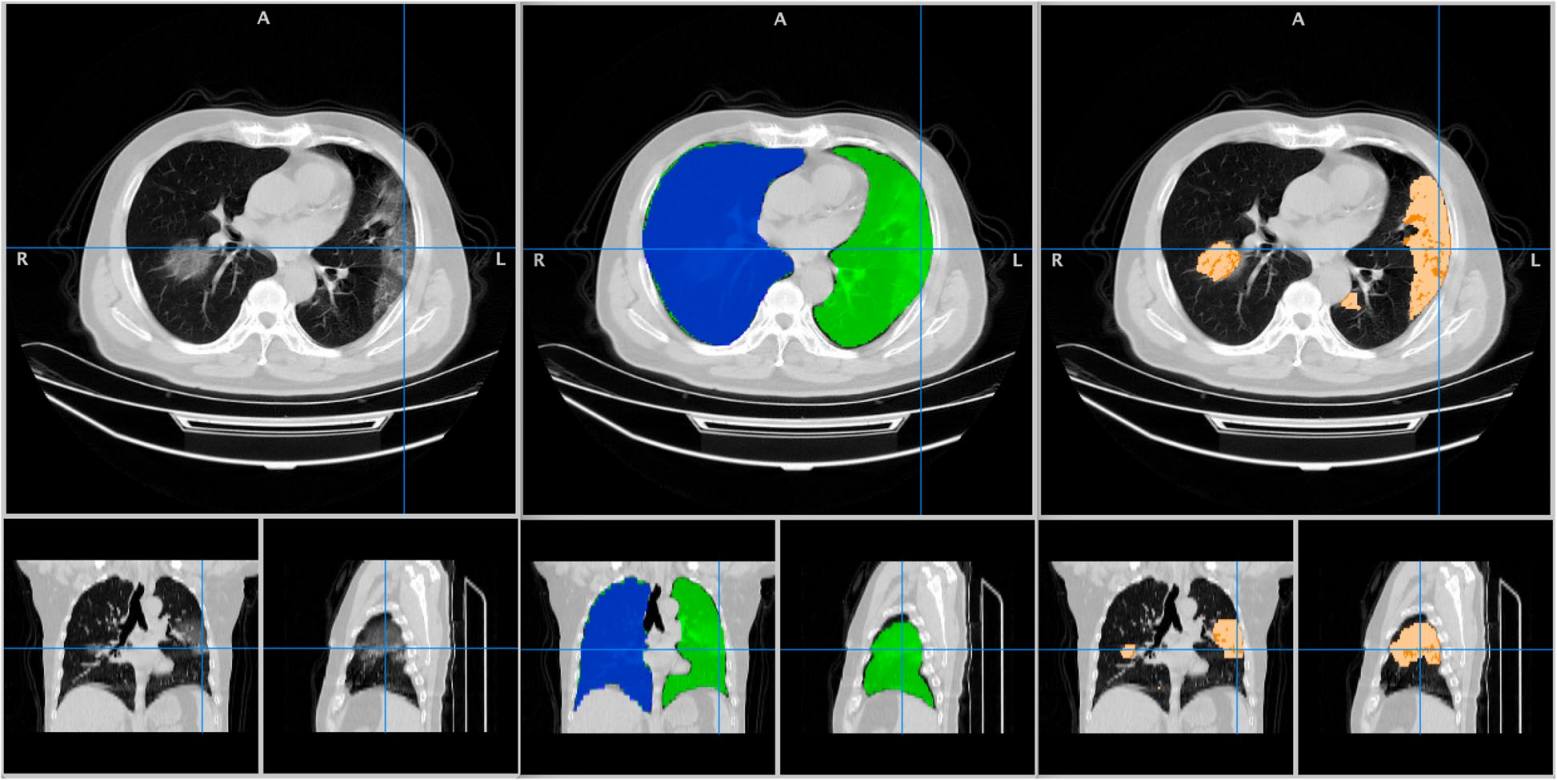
The output of *LungQuant* for a lung computed tomography scan. Left: original input image with axial, coronal and sagittal projections. Center: lung mask produced by *LungQuant* with different labels for the right and left lungs. Right: lesion masks produced by *LungQuant* for the ground-glass opacities (light orange) and the consolidations (dark orange)
